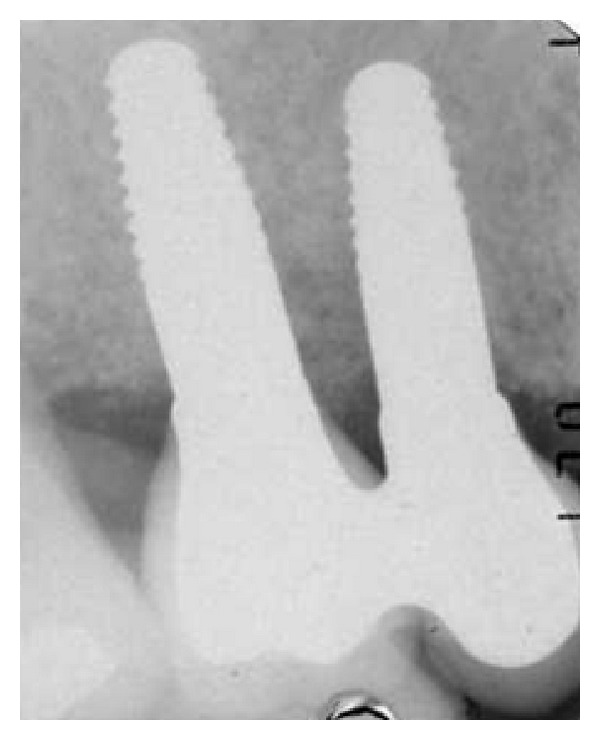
X-ray picture 48 months after surgery. No differences in marginal bone level changes can be observed from 12 to 48 months after surgery.
